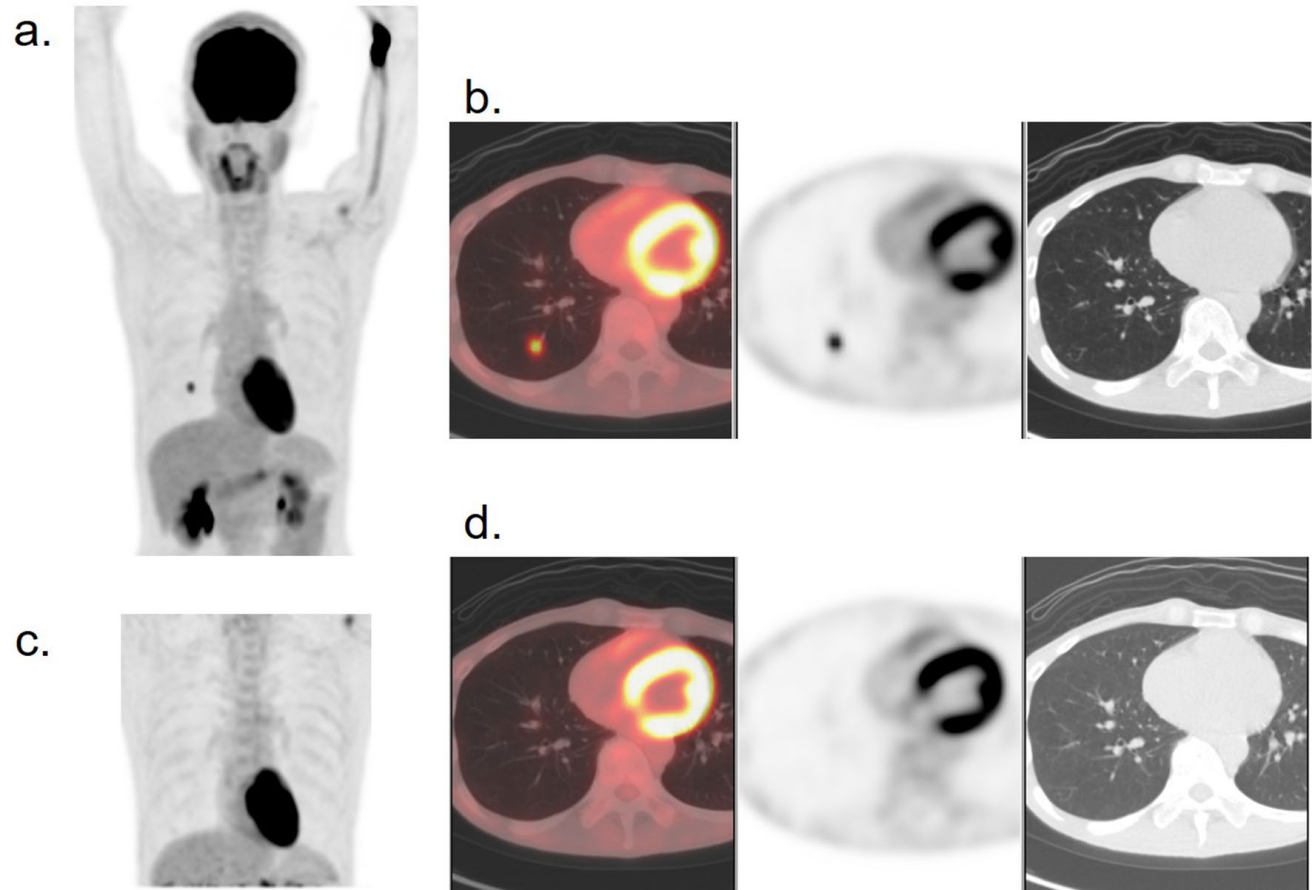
Transient hot clot in the right lung, which was considered to be a microembolism due to tracer extravasation, without abnormality on CT in the corresponding site a MIP, b axial images of fusion image (left), PET (middle) and CT (right)). The hot clot rapidly disappeared on the delayed scan that was performed 30 min later c MIP image, d axial image)
